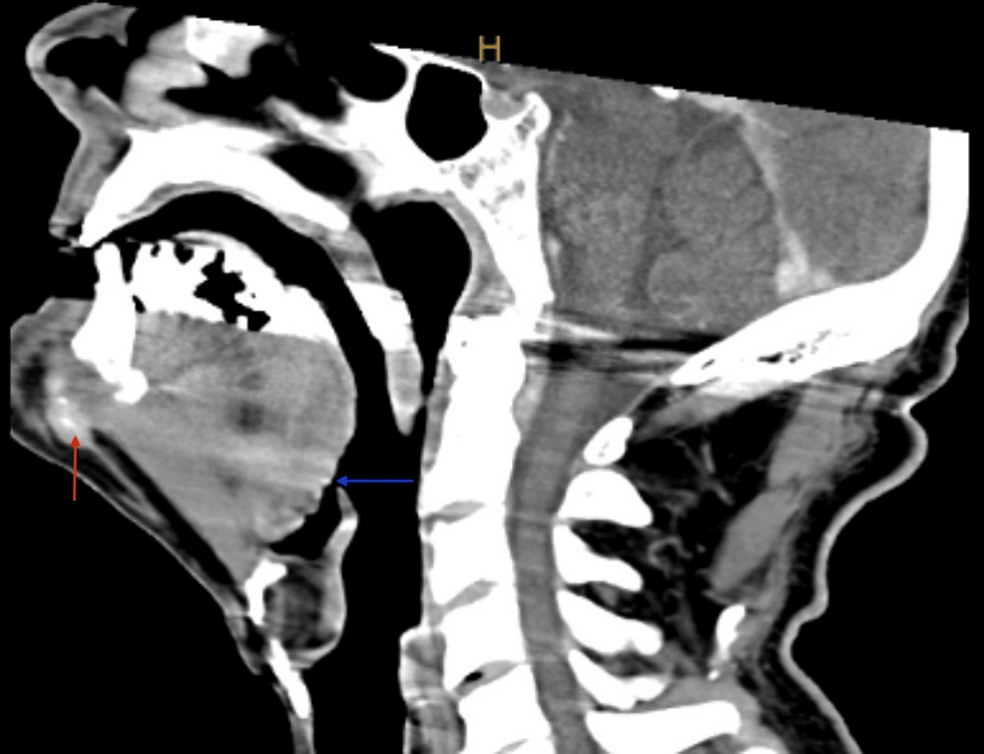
Post-induction Chemotherapy Sagittal CT Neck of Mandibular and Base of Tongue Mass Post induction sagittal CT neck with contrast showing worsening of soft tissue mass in the mandibular symphysis (red arrow) and decreased size of the left base of tongue mass (blue arrow)
